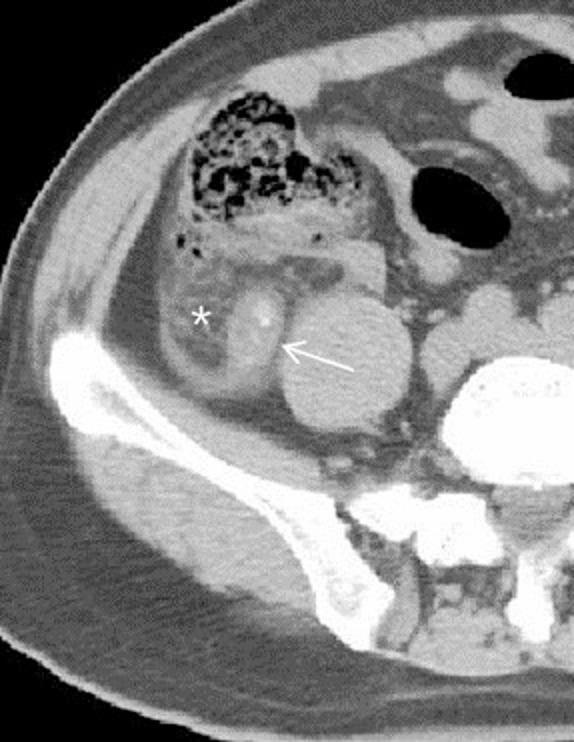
Moderate–severe fat stranding. Axial CT images show an enlarged appendix with appendicolith (arrow) and moderate–severe fat stranding (asterisk)
